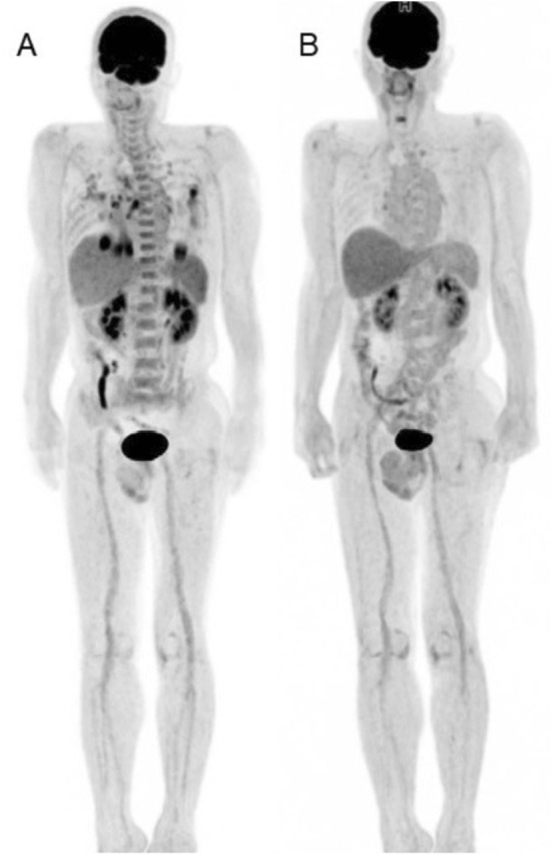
Whole-body [18]F-labeled fluorodeoxyglucose (FDG)-PET image of COVID-19. A Whole-body FDG-PET image of the patient with COVID-19 pneumonia (4 weeks after symptom onset and 3 weeks after negative RT-PCRs). B Whole-body FDG-PET image of the patient with COVID-19 pneumonia (4 weeks from previous FDG-PET scan). Intense FDG uptake was confirmed in lung lesion and mediastinal lymph node in the first image. In addition, increase FDG was seen in bone marrow and spleen. FDG uptake in the lung lesion and mediastinal lymph node disappeared, and uptake in the bone marrow and spleen decreases as physiological uptake level (Reproduced from Minamimoto et al*.* [54] under open copyright policy of the journal)
